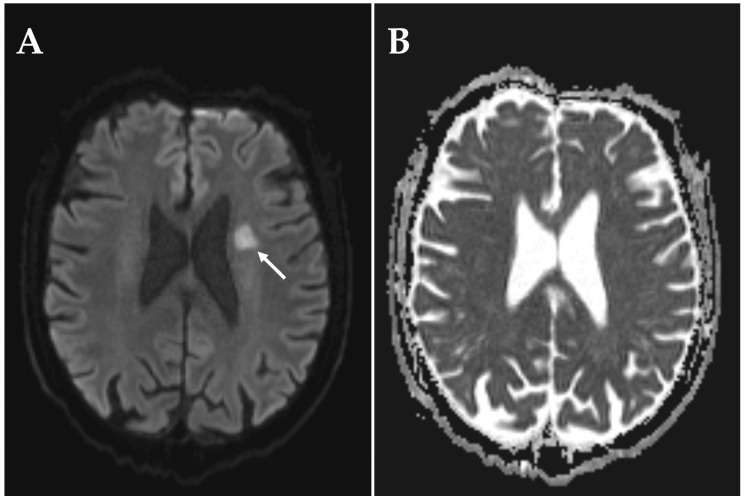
MRI sequences showing a subacute infarction in a patient with left-sided NAION. MRI was performed on a 3 T scanner within one day following onset of visual impairment. (A) DWI showing a subcortical hyperintense signal in the territory of the left middle cerebral artery. (B) ADC showing a normal signal in the corresponding region.
